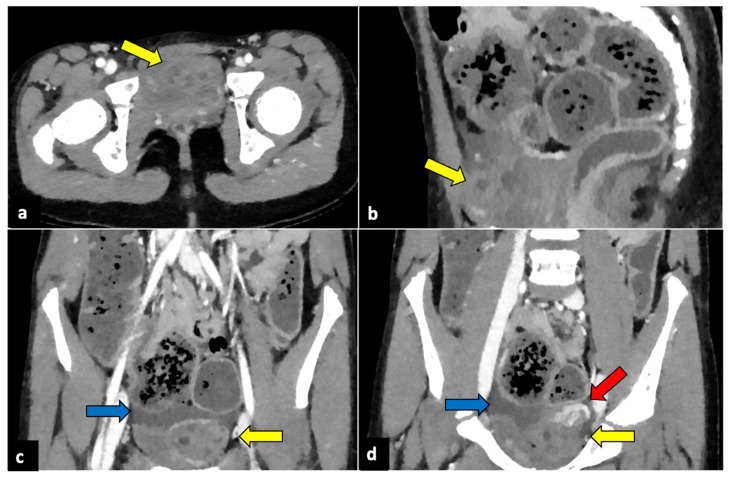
Left ovarian torsion in a 12-year-old woman with acute lower abdominal pain and vomiting. Axial (a), sagittal (b), and coronal (c,d) contrast-enhanced CT images show enlarged and oedematous left adnexa migrated to the midline and anteriorly to the uterus (yellow arrows), with peripherally displaced ovarian follicles. A small amount of free pelvic fluid (blue arrows) is associated. On the coronal plane, the twisted and oedematous ovarian vascular pedicle (red arrow) can be easily detected.
